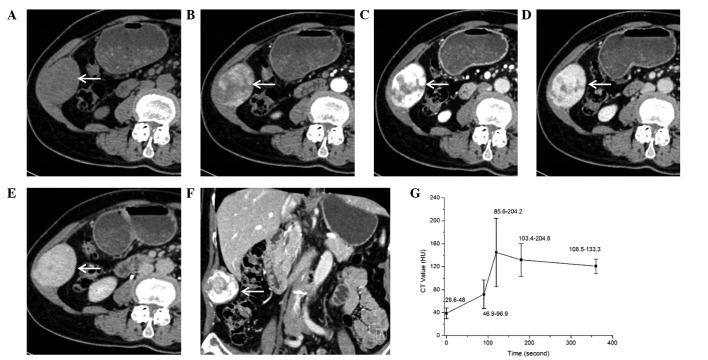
Computed tomography (CT) imaging findings of the schwannoma. (A) Conventional CT scan revealing an oval mass (white arrow) in the right lower abdominal wall. Contrast-enhanced CT scan showing a gradually- and heterogeneously-enhanced lesion with high density in (B) the arterial phase, (C) the venous phase and (D and E) the delay phase at different times. (F) Coronal image showing that the mass is in the abdominal wall. (G) The time-intensity curve displaying the characteristics of enhancement following agent injection.
